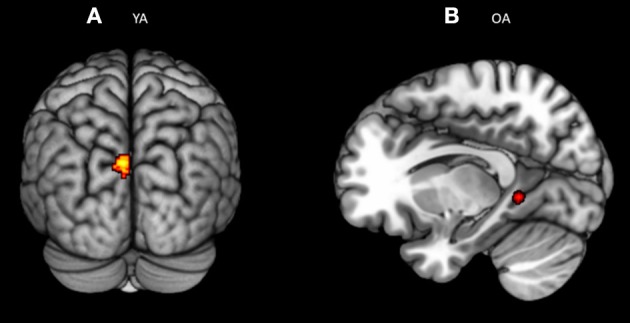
Significant blood-oxygen-level dependent (BOLD) signal for (A) young bilinguals and (B) older bilinguals for the executive control contrast (Incongruent – Congruent). Statistical parametric maps overlaid on the average T1-weighted anatomy of all subjects (*p* < 0.001 and *K* > 20).
